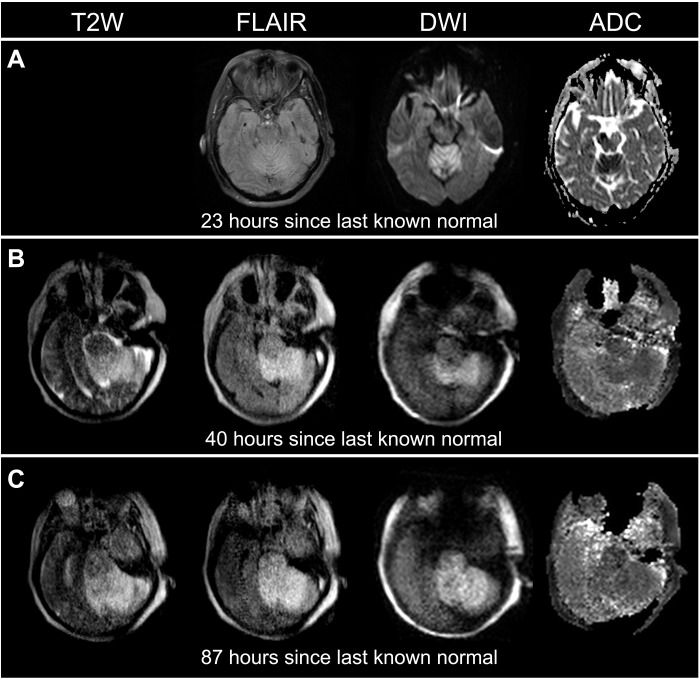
Serial imaging with pMRI facilitated dynamic monitoring of acute ischemic stroke progression in a 66-year-old female with a brainstem stroke. (A) The SOC MRI (3 T) was obtained 23 hours since last known normal. (B) The first pMRI exam was obtained 40 hours since last known normal. (C) The second pMRI exam was obtained 87 hours since last known normal. Serial pMRI exams (B and C) revealed an evolution and extension of cerebral infarction from the cerebellum into the brainstem (70.430 to 97.681 cm^3^). The SOC MRI exam did not include a T2W sequence.
